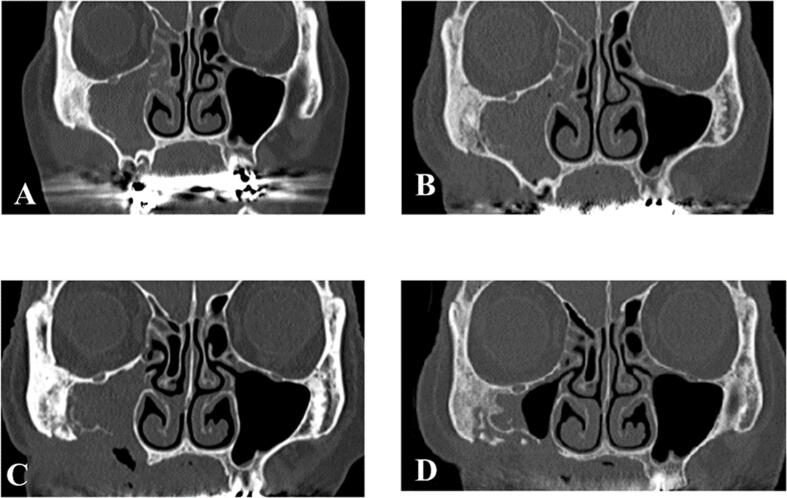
Computed tomography findings (coronal sections). A: Initial visit. B: 1 month later. C: 12 months later. D: 24 months later.
